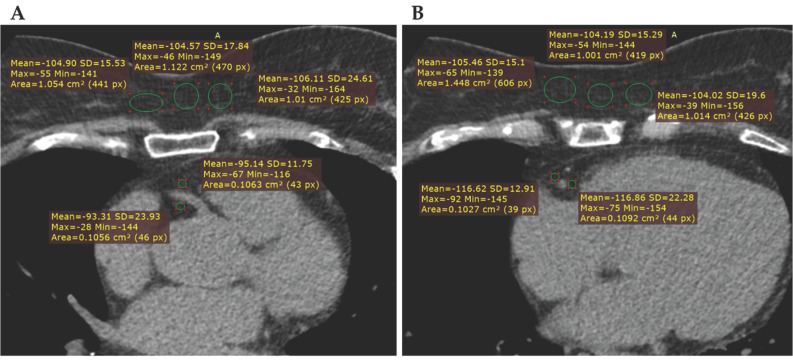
Showing two axial non-enhanced CT images, with examples for ROI placement (green circles) in the RCA pericoronary adipose tissue and subcutaneous adipose tissue at the aortic root level (A) and the four-chamber view level (B). The adjacent yellow annotations show the ROI-derived attenuation parameters, including mean attenuation in Hounsfield units (HU), standard deviation (SD), maximum and minimum HU values, and ROI area in cm^2^/pixels.
